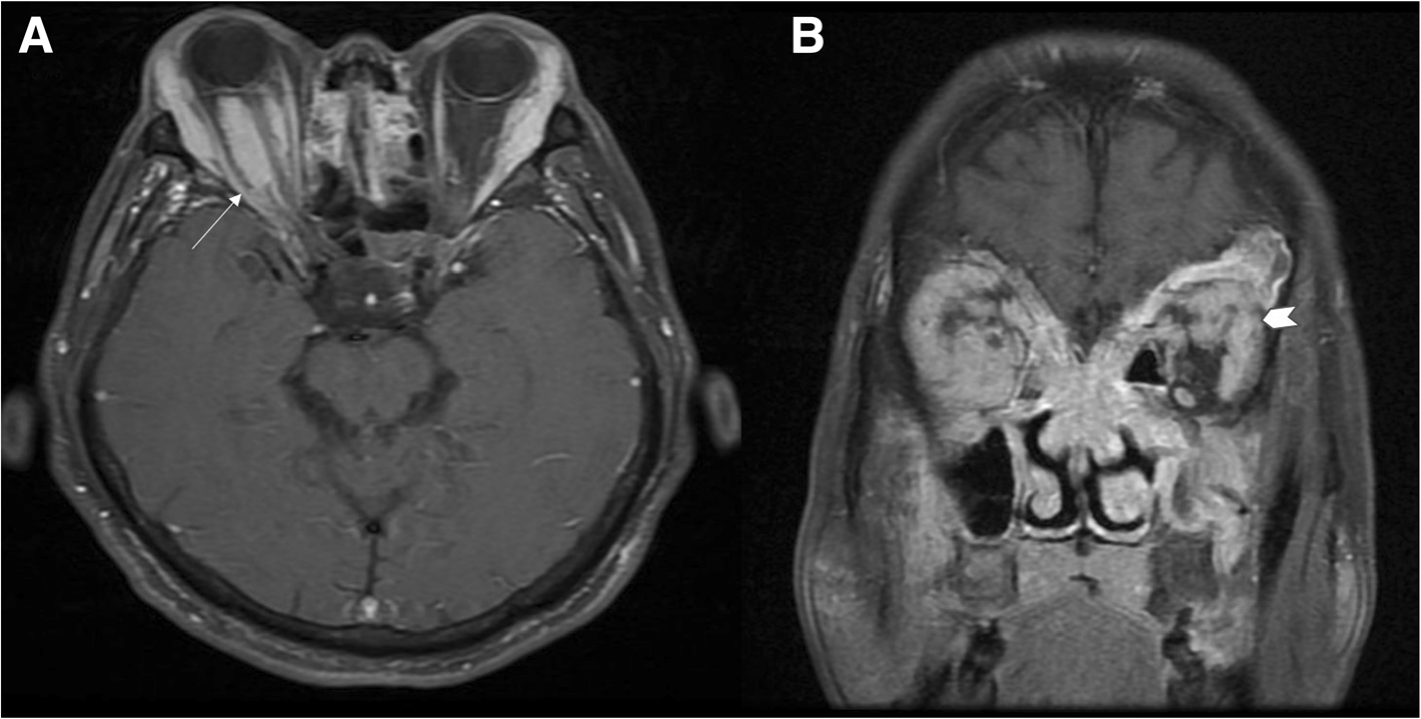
Optic nerve was compressed by pseudotumor masses. Axial and coronal contrast enhanced T1-weighted MRI showing pseudotumor mass circles of optic nerve, was compressed by pseudotumor masses (white arrow) (a). Extraocular muscles appeared enlarged as masses and optic nerves were oppressed (white arrowhead) (b)
